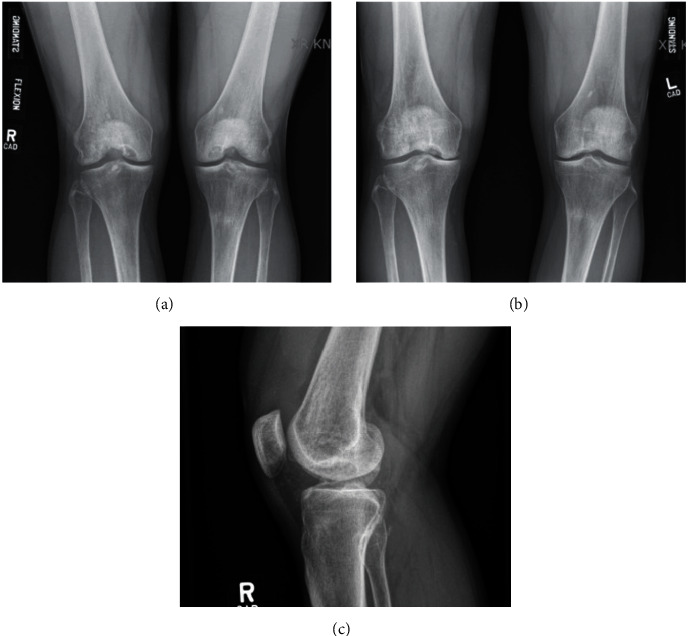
Preoperative radiographs. (a) Posterior-anterior weight-bearing view. (b) Anterior-posterior weight-bearing view. (c) Lateral view.
